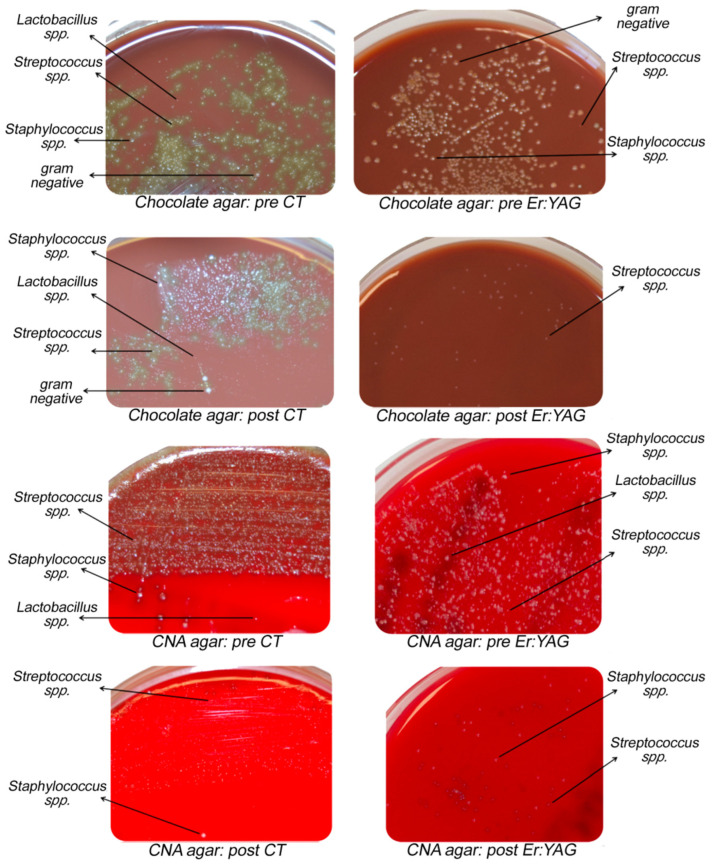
Difference in gram-positive and gram-negative colonies growth after Er:YAG treatments (right) vs. CT (left). Pre CT: pre-treatment with conventional therapy; post CT: post-treatment with conventional therapy; pre Er:YAG: pre-treatment with laser therapy; post Er:YAG: post-treatment with laser therapy.
